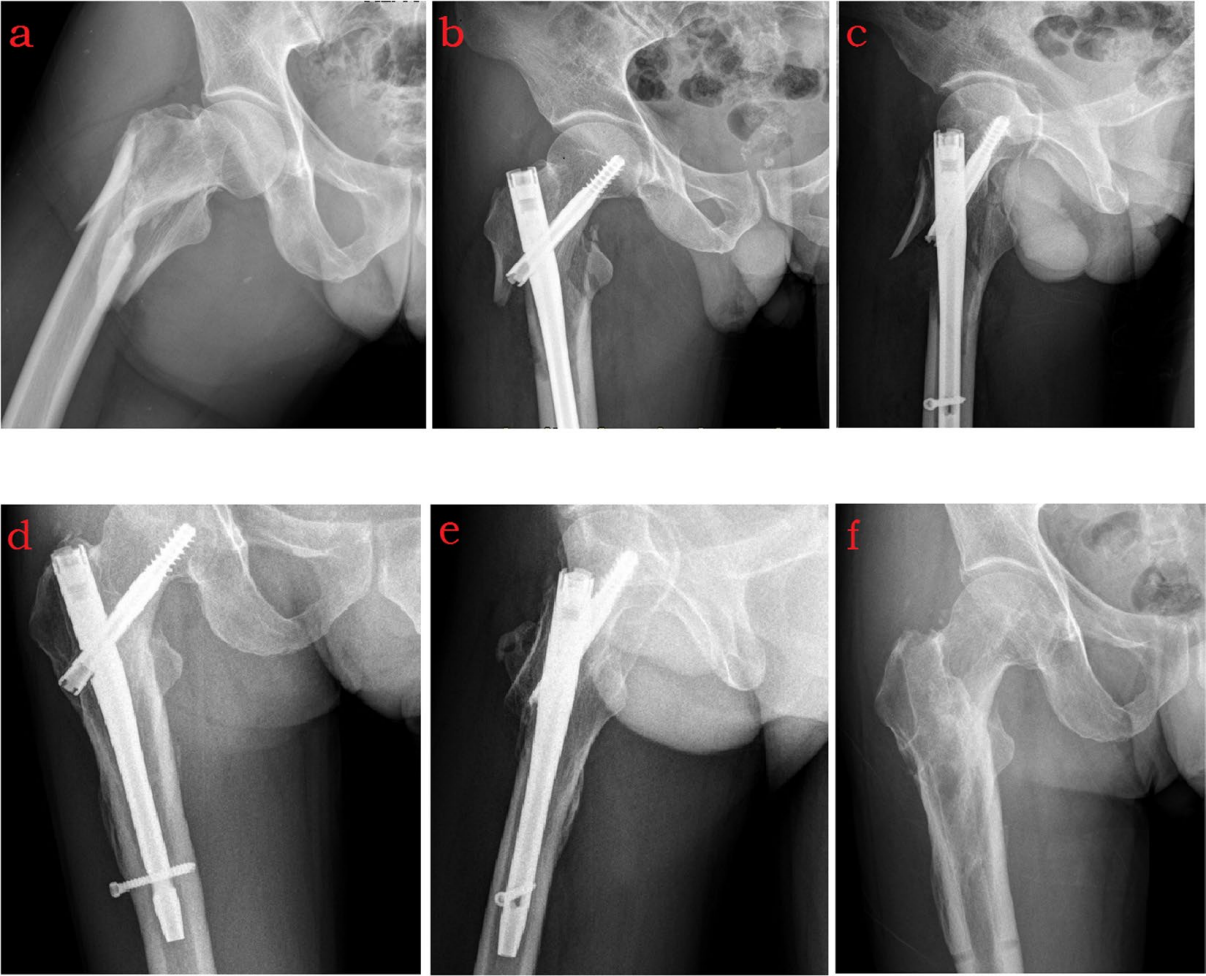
A 38-year-old male with right intertrochanteric fracture fixed with Gamma nail. (a) Radiograph of pelvis showed the fracture of right intertrochanteric, with broken lateral wall. (b,c) Anteroposterior and oblique plain film of right hip taken 2 weeks after surgery showed significant lateral wall defect. (d,e) Fracture healing was showed 6 months after surgery.
